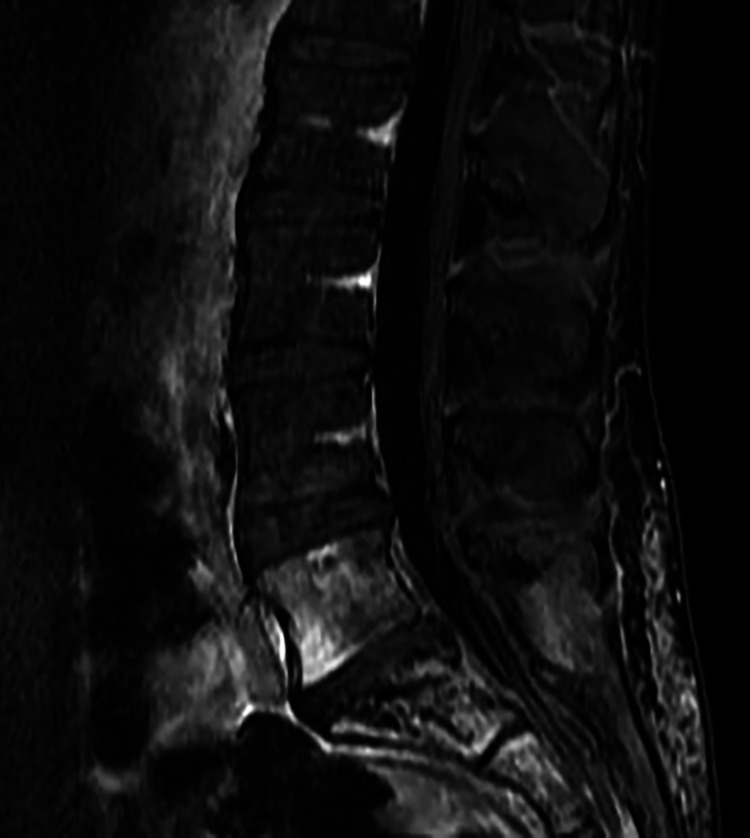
Sagittal T1-weighted fluid-attenuated inversion recovery (FLAIR) MRI with contrast of the lumbosacral junction Sagittal T1-weighted fluid-attenuated inversion recovery (FLAIR) MRI with contrast of the lumbar spine demonstrating fatty replacement at the L5 vertebral body and sacrum (red arrows) with postoperative scarring within in the subcutaneous tissues, paraspinal muscles, and sacrum (blue arrows).
